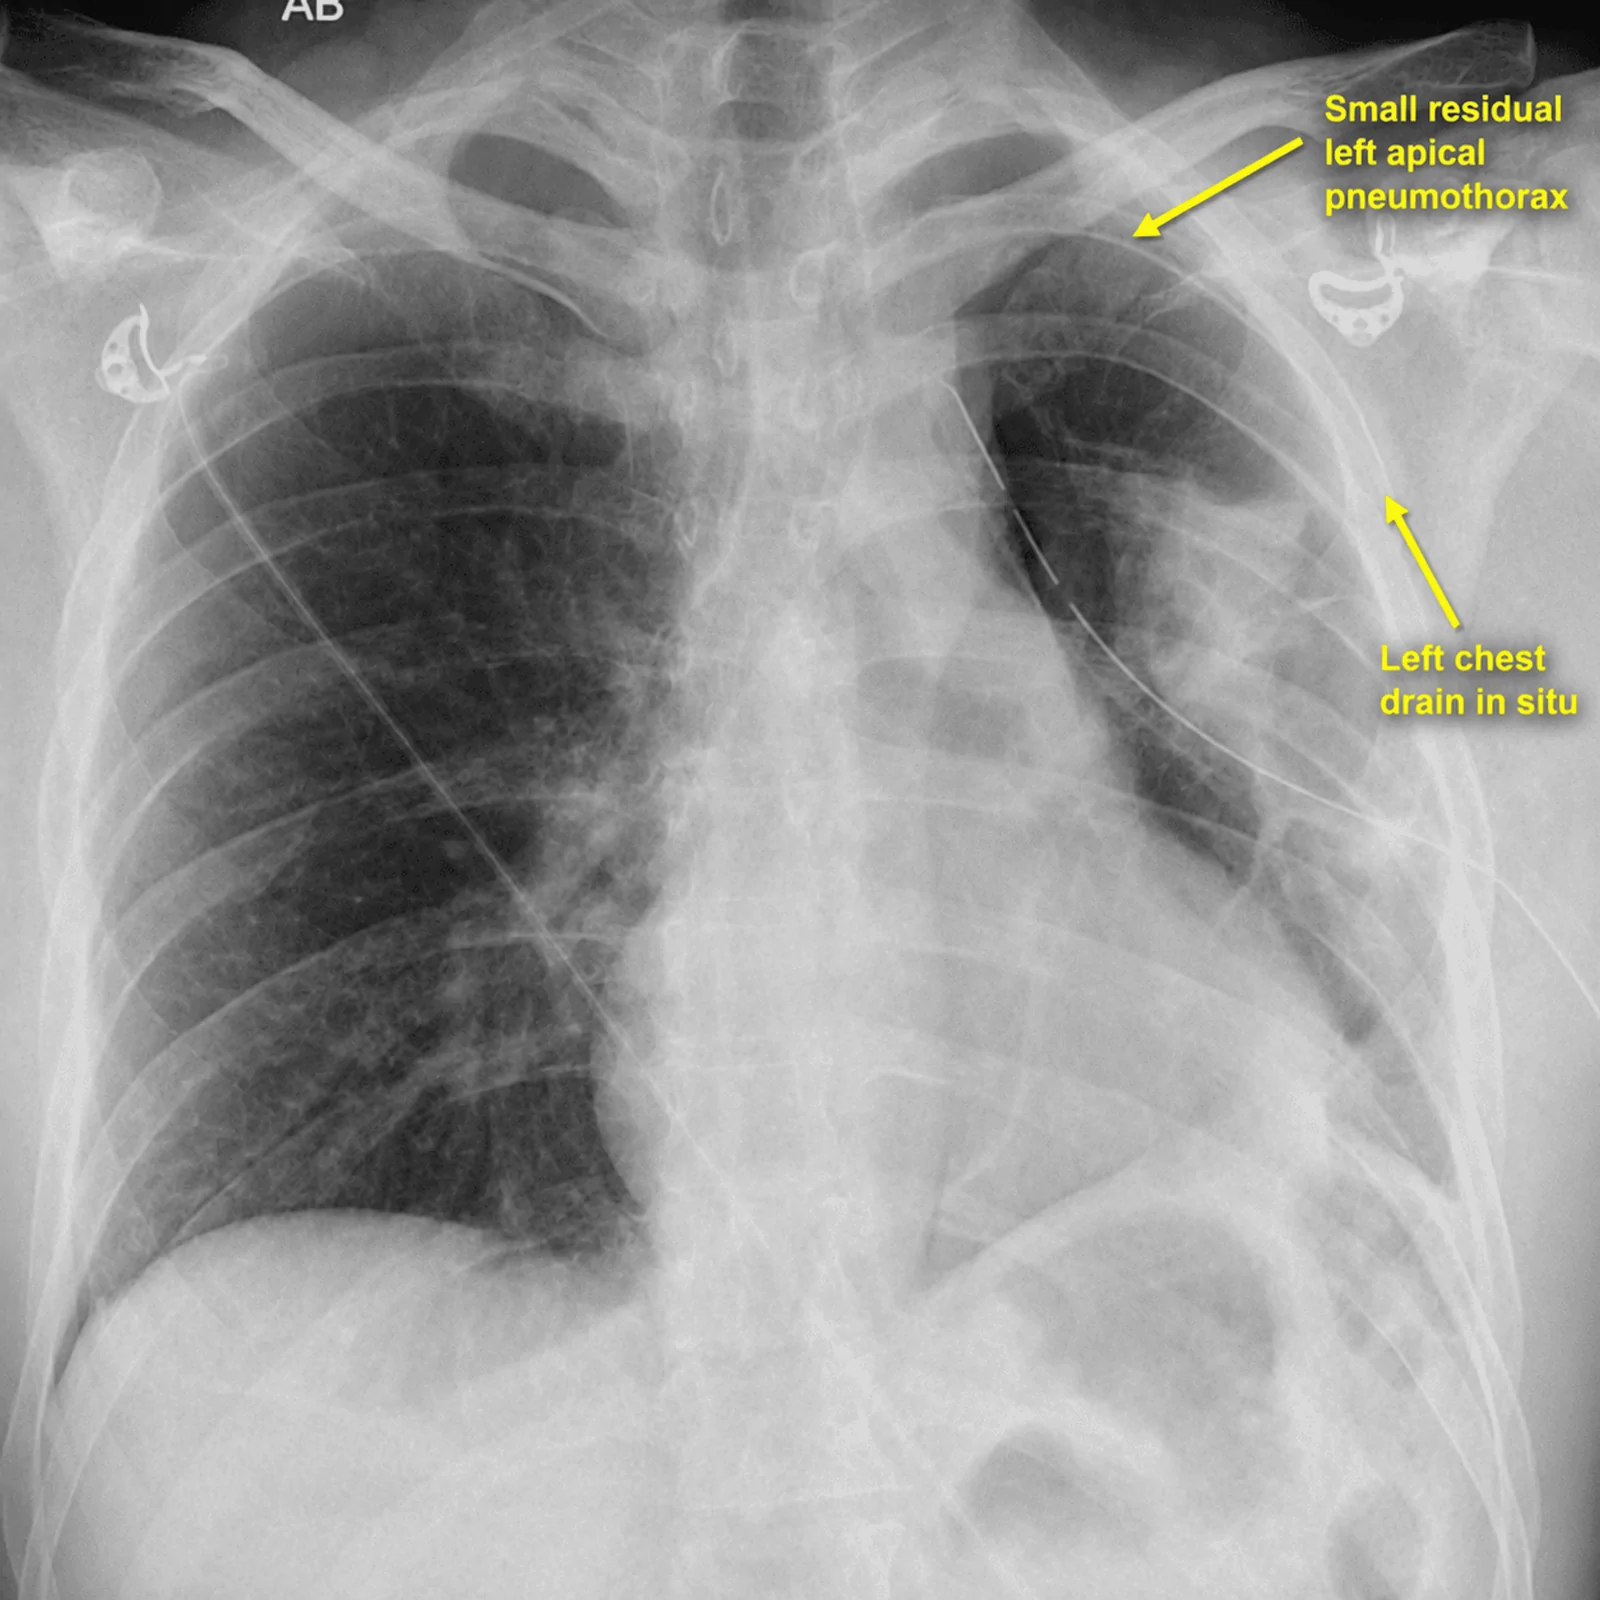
Post-drain chest radiograph: interval re-expansion of the left lung following insertion of the left intercostal chest drain, with a small residual left apical pneumothorax The annotations indicate the residual pleural air and chest-drain position.
